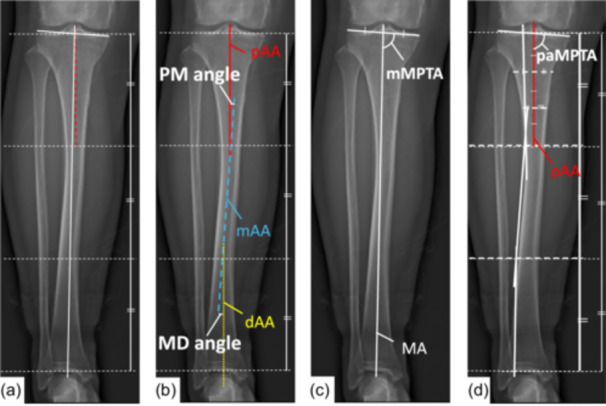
Method for radiographic analysis of tibial alignment. (a) The tibia is divided into three equal segments by horizontal lines. (b) Anatomical axes are defined for each segment: proximal (pAA), middle (mAA), and distal (dAA). Deformity angles are measured between these axes: the proximal‐middle (PM) angle and the middle‐distal (MD) angle. (c) The mechanical medial proximal tibial angle (mMPTA) is measured using the mechanical axis (MA), which connects the center of the tibial plateau to the center of the talus. (d) The proximal anatomical medial proximal tibial angle (paMPTA) is measured using the proximal anatomical axis (pAA).
